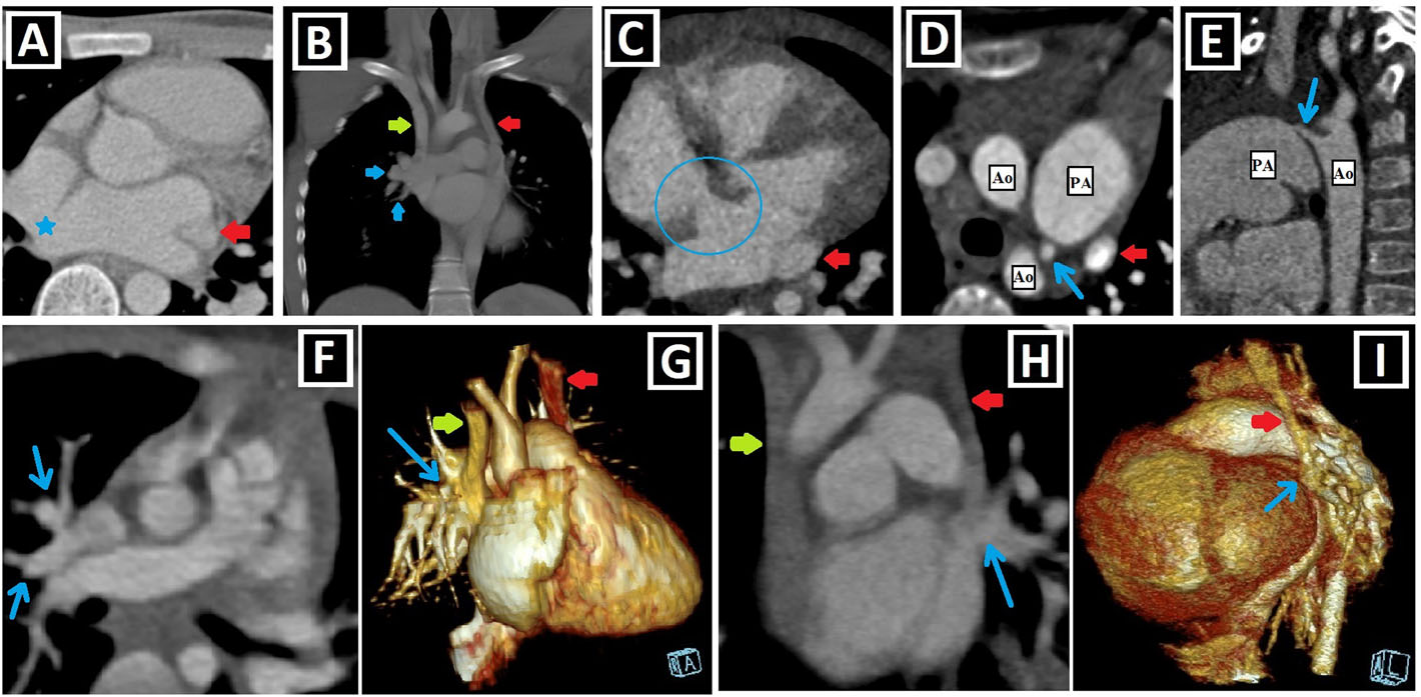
Shunt lesions accompanying PLSVC-1. a, b Axial (a) and coronal-oblique reformatted (b) CT images indicate sinus venosus ASD (blue star) in a patient with PLSVC (red arrow) and accompanying abnormal right upper pulmonary venous drainage (blue arrows) to RSVC (green arrow). c Axial CT image depicts the ostium secundum ASD (blue circle) in a patient with PLSVC (red arrow). d, e Axial (d) and sagittal-oblique reformatted (e) CT images depict the accompanying PDA (blue arrows) in a patient with PLSVC (red arrow). PA represents the pulmonary artery, and Ao represents aorta. f, g Axial (f) and 3D VRT (g) CT images indicate the PAPVD to the RSVC (blue arrows) in a patient with double SVC (green and red arrows). h, i Coronal-oblique reformatted (h), and 3D VRT reconstructed (i) CT images indicate the PAPVD to the PLSVC (blue arrows) in a patient with double SVC (green and red arrows)
